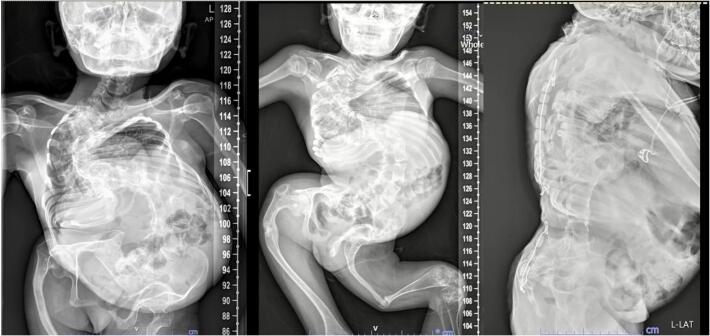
Whole spine X-ray showing diffuse osteopenia and severe scoliosis with right thoracic and left lumbar convexities, causing relatively decreased volume of the right hemithorax and abnormal thoracic cage.
